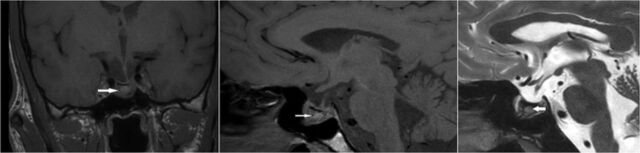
Magnetic resonance imaging of sellar region. T1-weighted coronal (left panel) and sagittal (middle panel) and T2-weighted sagittal (right panel) images show macroadenoma (arrows) with stalk deviation toward the left side. The lesion is hyperintense on T1-weighted images and hypointense on the T2-weighted image, suggestive of hemorrhage within.
